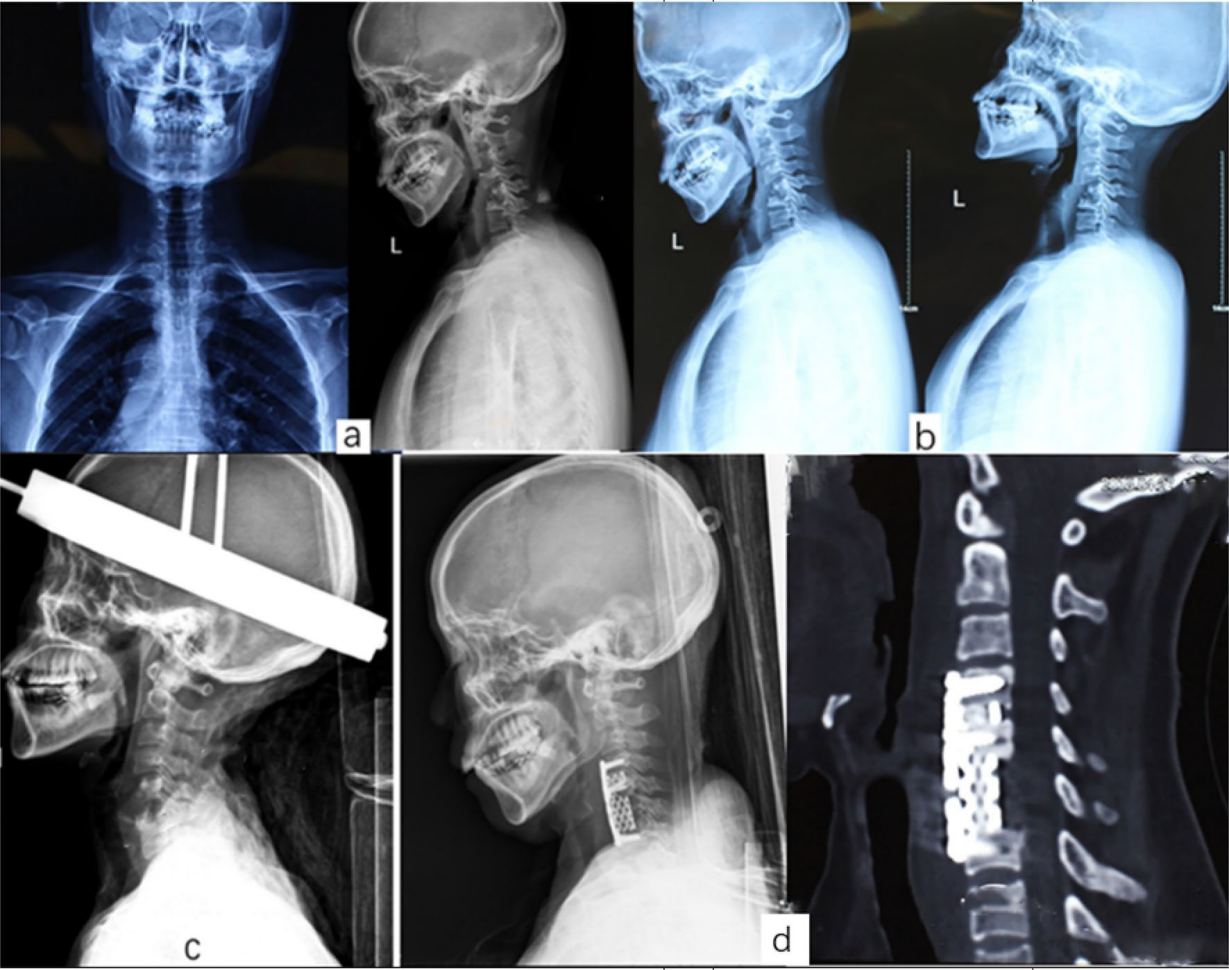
A 63 years old female with C5–6 TB infection with refractory kyphosis who underwent preoperative skull traction (a) preoperative AP and lateral X-ray films, (b) dynamic X-ray showing no obvious change in kyphosis in both flexion and extension, (c) after 8 days of halo-traction the cervical lordosis was recovered, (d) postoperative X-ray and CT-scan at final follow-up showing good bone fusion and lordosis restoration.
